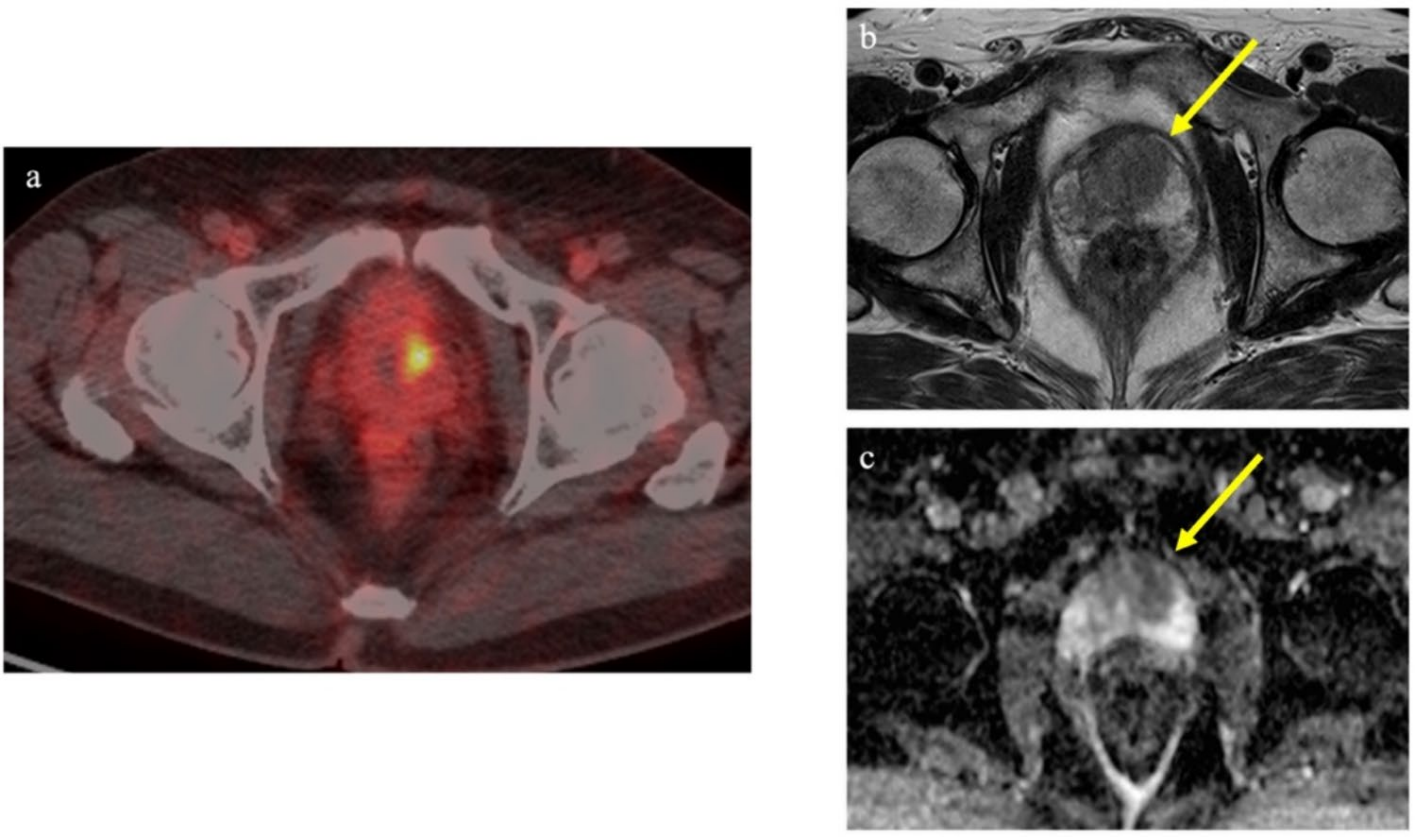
PET/CT using ^18^F-FSU-880 in a 70-year-old man with biochemical recurrence after radiation therapy (cT3aN0, Gleason score 4 + 5). PSA level increased to 2.33 ng/ml. Focal uptake was observed in the transitional zone of the prostate on the left side, suggesting local recurrence (a). The initial MRI (conducted 8 years earlier) showed a signal-hypointense lesion on T2-weighted imaging with restriction of water diffusion in the transitional zone on the left side, at the same site as the recurrence. a Transaxial PET/CT fusion image. b Transaxial T2-weighted MR image. c Transaxial ADC map
